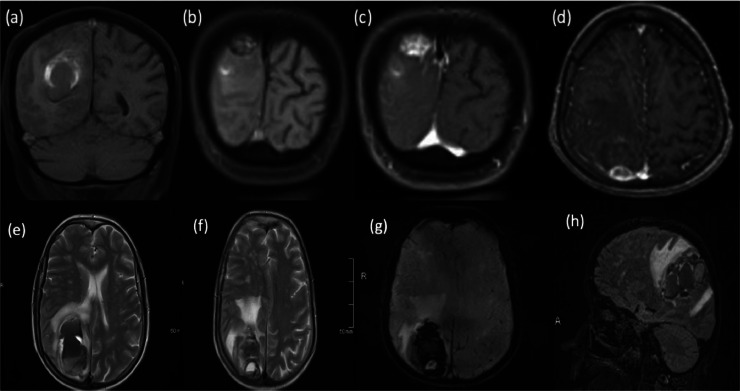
Multi-sequential magnetic resonance images (MRI) of the brain show an angiomatous meningioma (AM) at the right parieto-occipital lobe. It shows isointense signal intensity in T1WI with peripheral high signal intensity (A and B), and heterogeneous enhancement in post-contrast images (C and D) on axial T2-weighted image (T2WI) (E and F), susceptibility-weighted image (SWI) (G), and sagittal fluid-attenuated inversion recovery (FLAIR) (H) show central hemorrhagic changes and an adjacent large intra-cerebral hematoma, which depicts variable signal intensities of variable blood ages on T1, T2, and FLAIR sequences and magnetic susceptibility artifacts on SWI. Blood–fluid levels within the lesion and the intracerebral hematoma indicate active bleeding.
